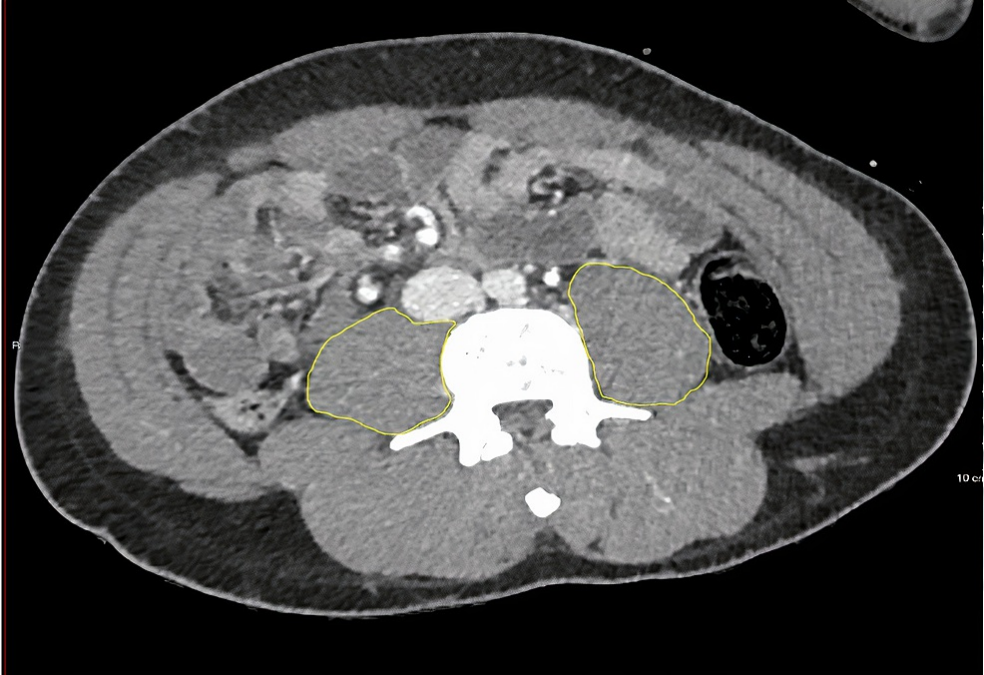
Axial CT image of total psoas area delineated in yellow at the level of the L3 vertebra
